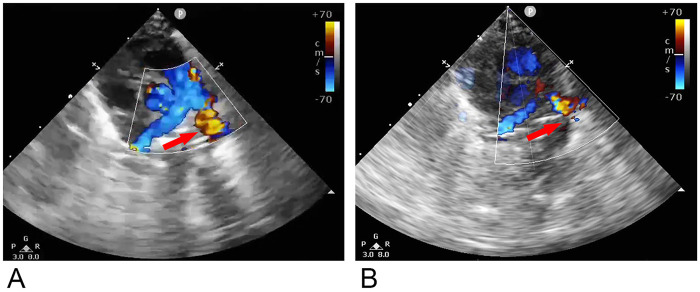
Echocardiography before and after PDA ligation. (A): Echocardiography performed 1 day prior to surgery revealed color Doppler evidence of multicolored blood flow within the pulmonary artery deriving from the descending aorta (red arrow); (B): Echocardiography conducted 2 h postoperatively demonstrated no residual shunt at the level of great artery (indicated by the red arrow).
